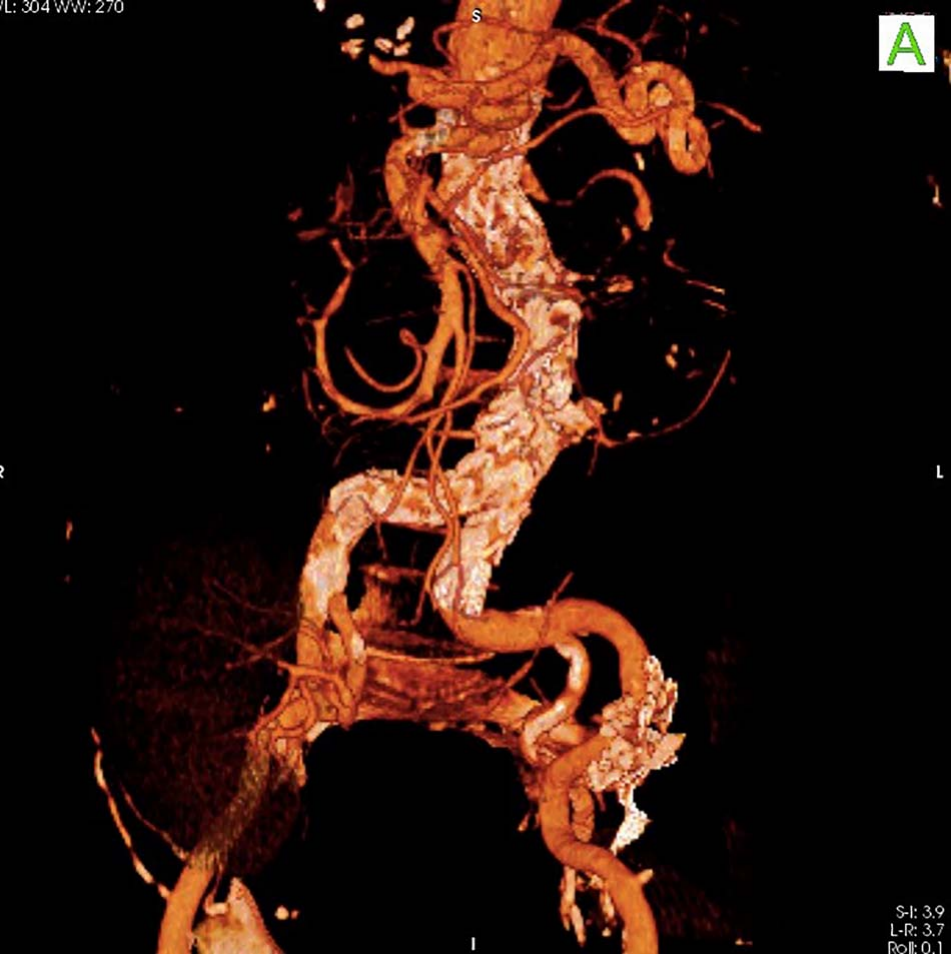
Follow-up CT angiography.
